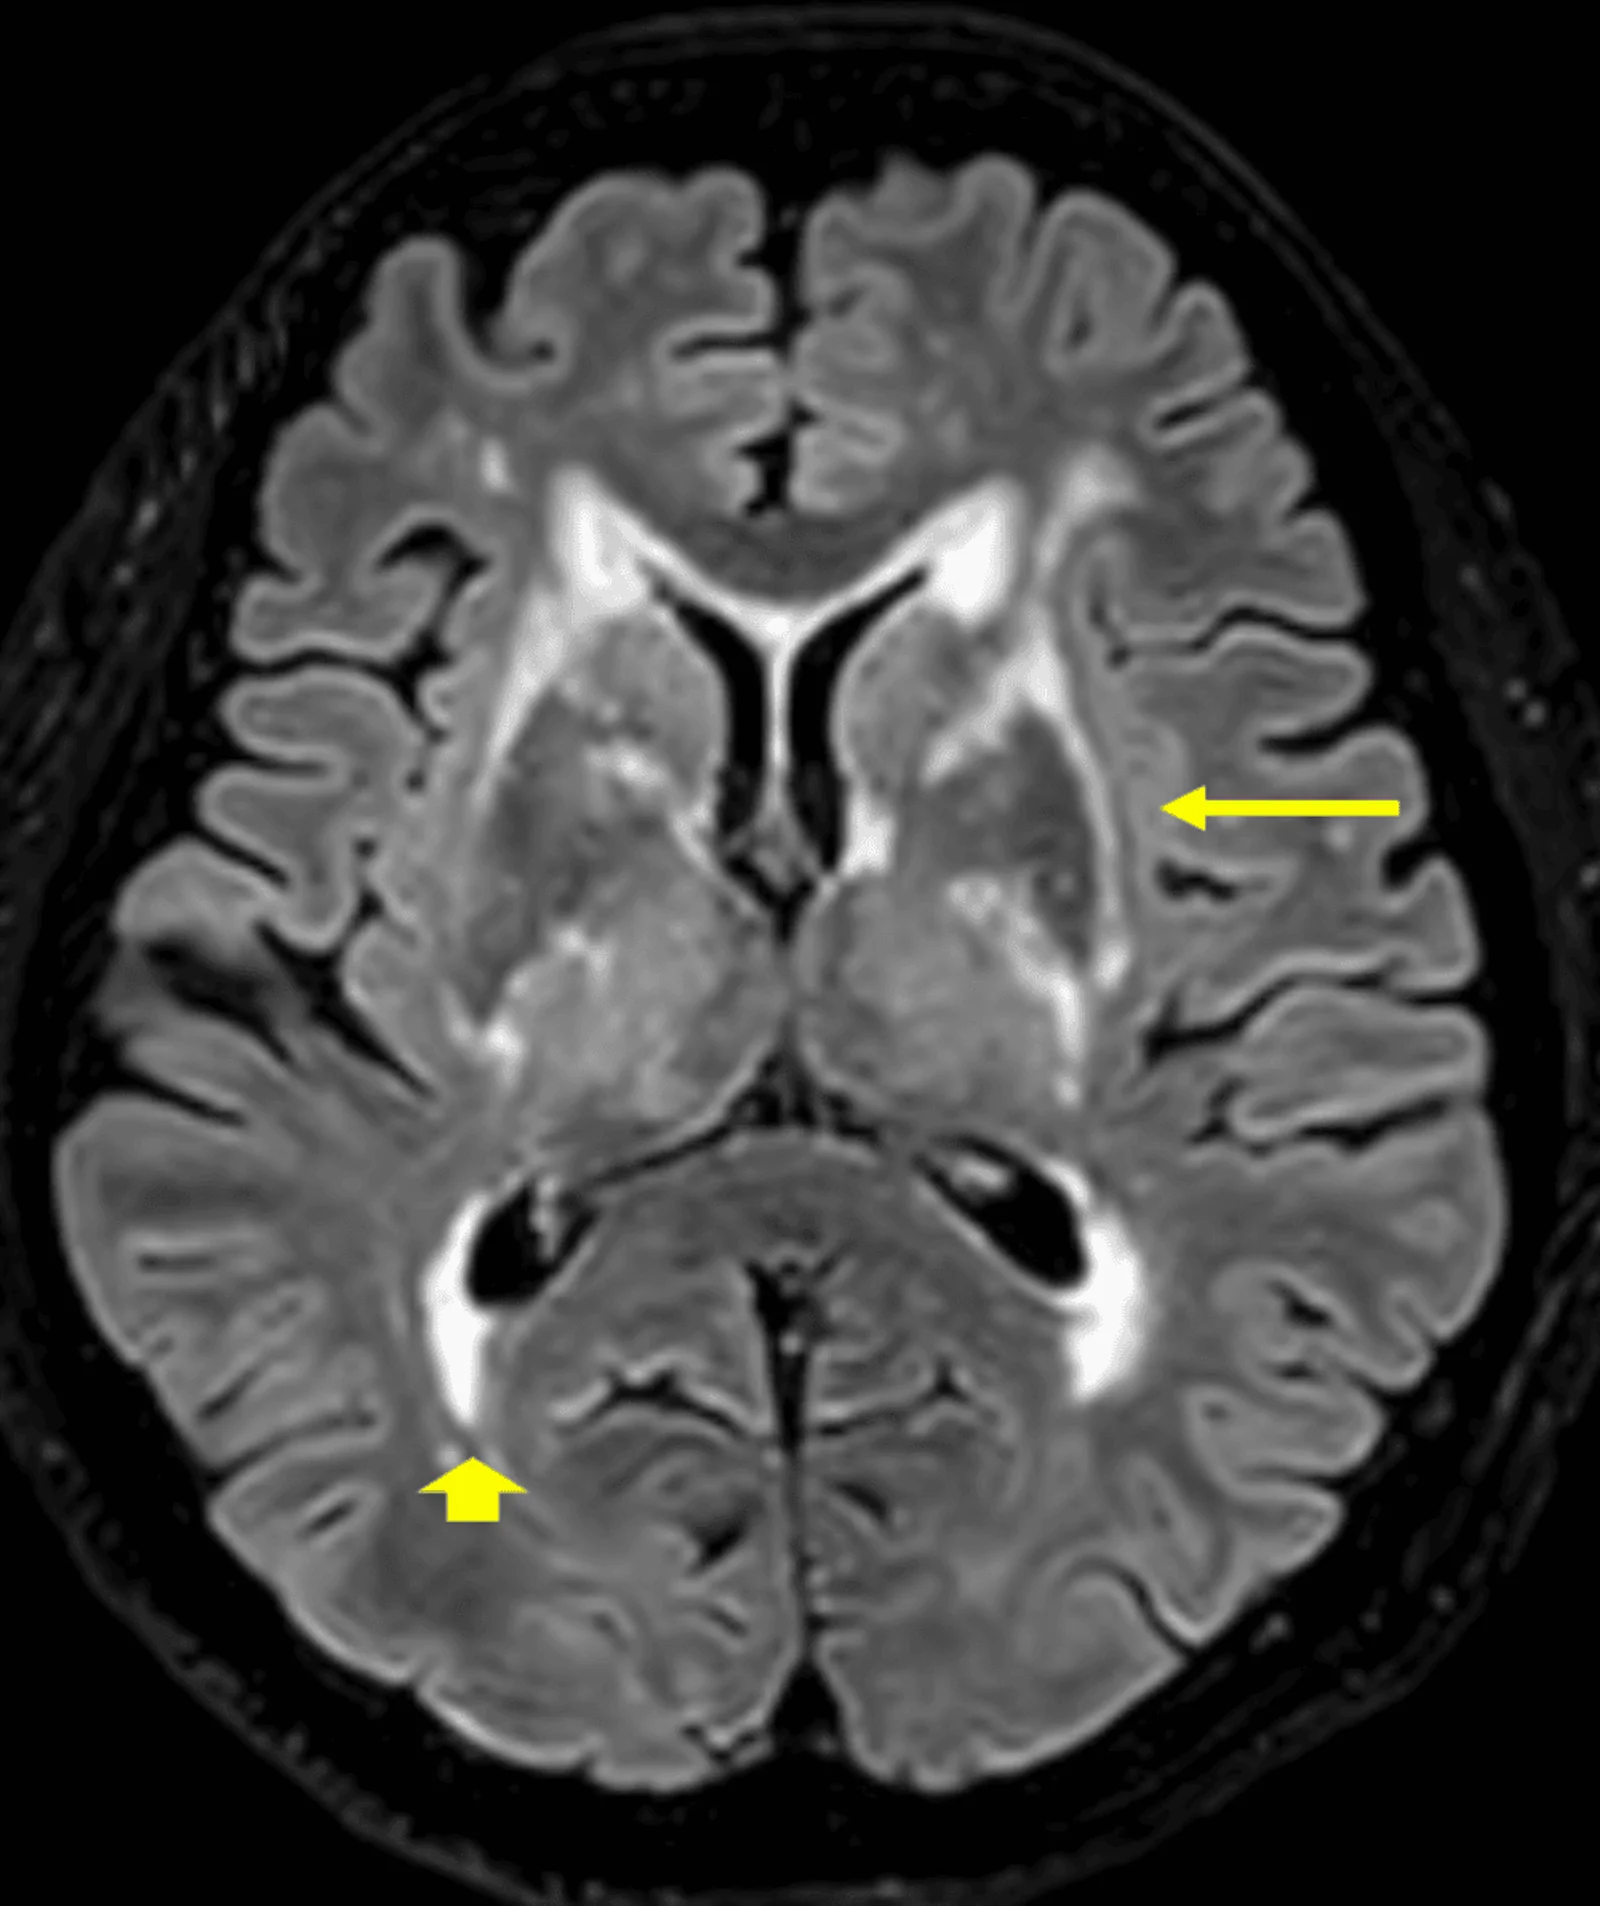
Axial brain MRI fluid-attenuated inversion recovery sequence at the level of the basal ganglia. Brain MRI showing extensive fluid-attenuated inversion recovery hyperintensities in the periventricular (short yellow arrow) and deep white matter, including bilateral external capsules (long yellow arrow).
